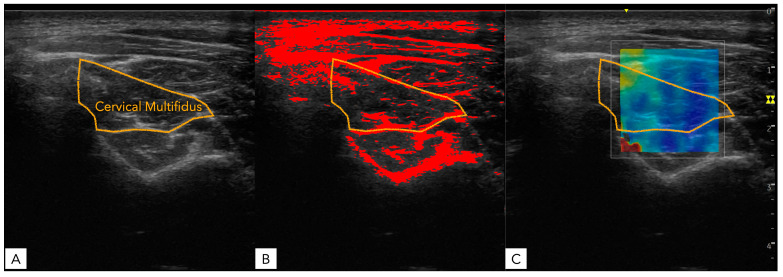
Ultrasound imaging of the cervical multifidus muscle acquired at the C4–C5 level: (A) raw image; (B) fat infiltration calculation; (C) shear wave elastography.
